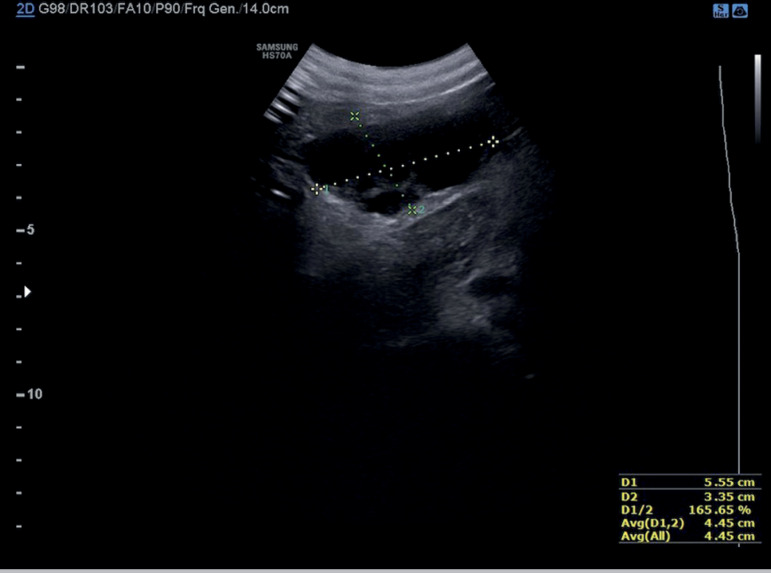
Transabdominal ultrasonography of the transposed right ovary.
